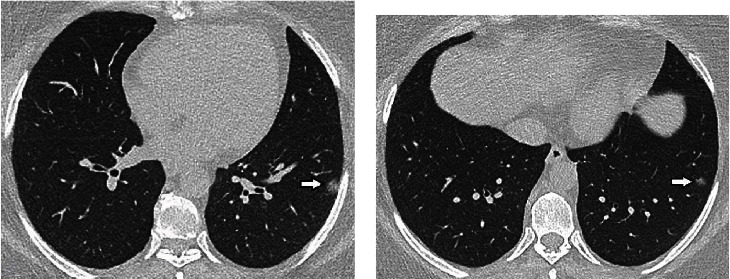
Radiological evaluation of nonsevere COVID-19 pneumonia in a patient with II genotype. A 47-year-old woman with a complaining of muscle ache had a positive RT-PCR test result for SARS-CoV-2. Axial nonenhanced CT scan of the chest ((a, b) axial images) shows areas of peripheral rounded ground-glass opacities in the left lower lobe (arrows) representing mild COVID-19 pneumonia.
